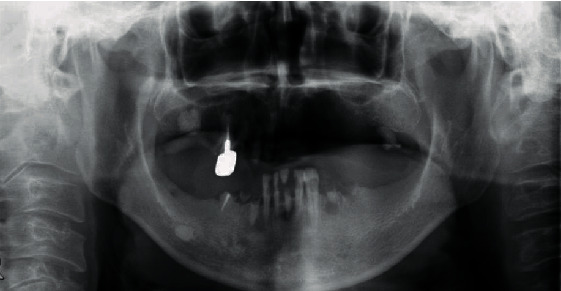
Panoramic X-ray photo. There are few teeth in his mouth, and he has difficulty eating.
